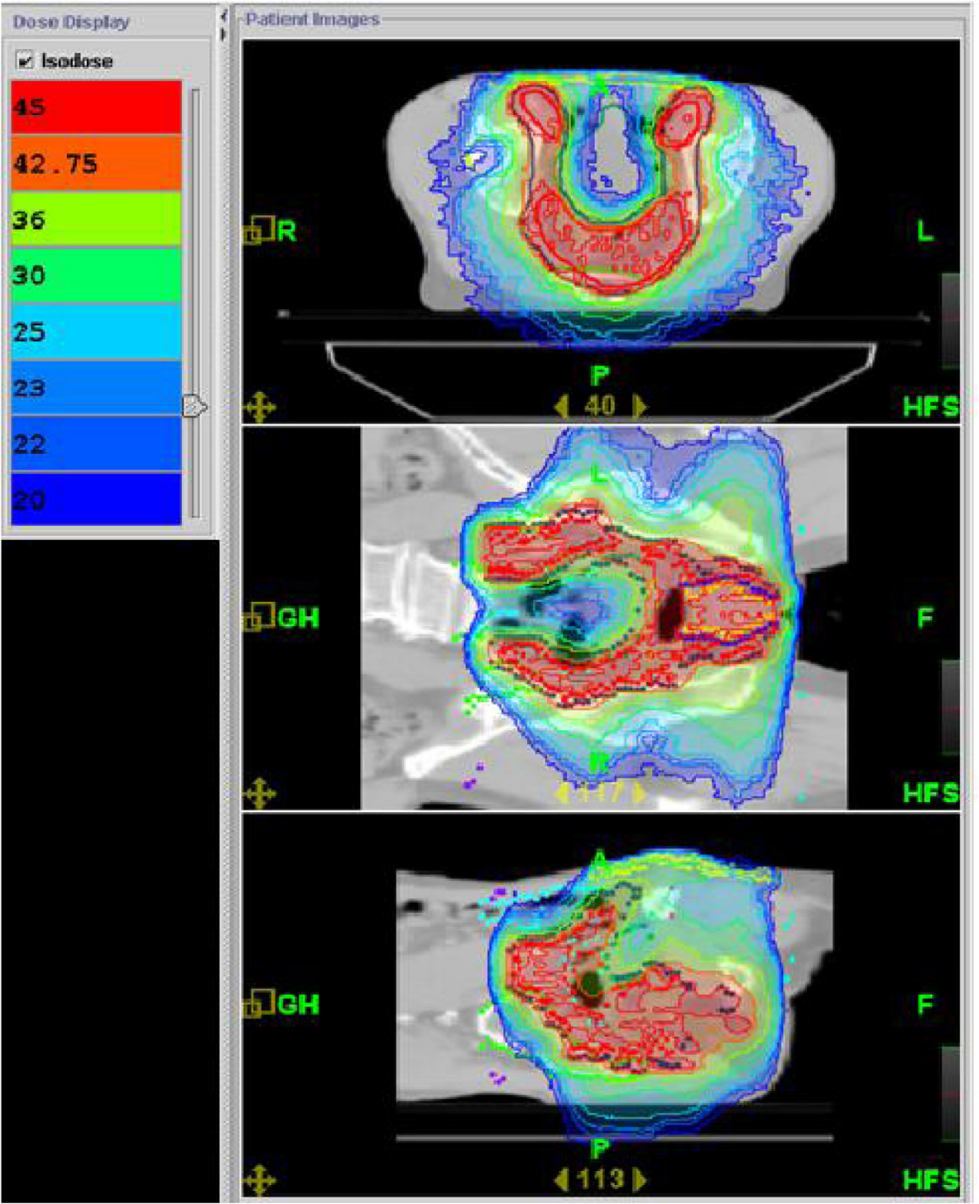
Dose distribution on planning CT with Tomotherapy for the first plan of treatment (45 Gy) for a patient with anal cancer
